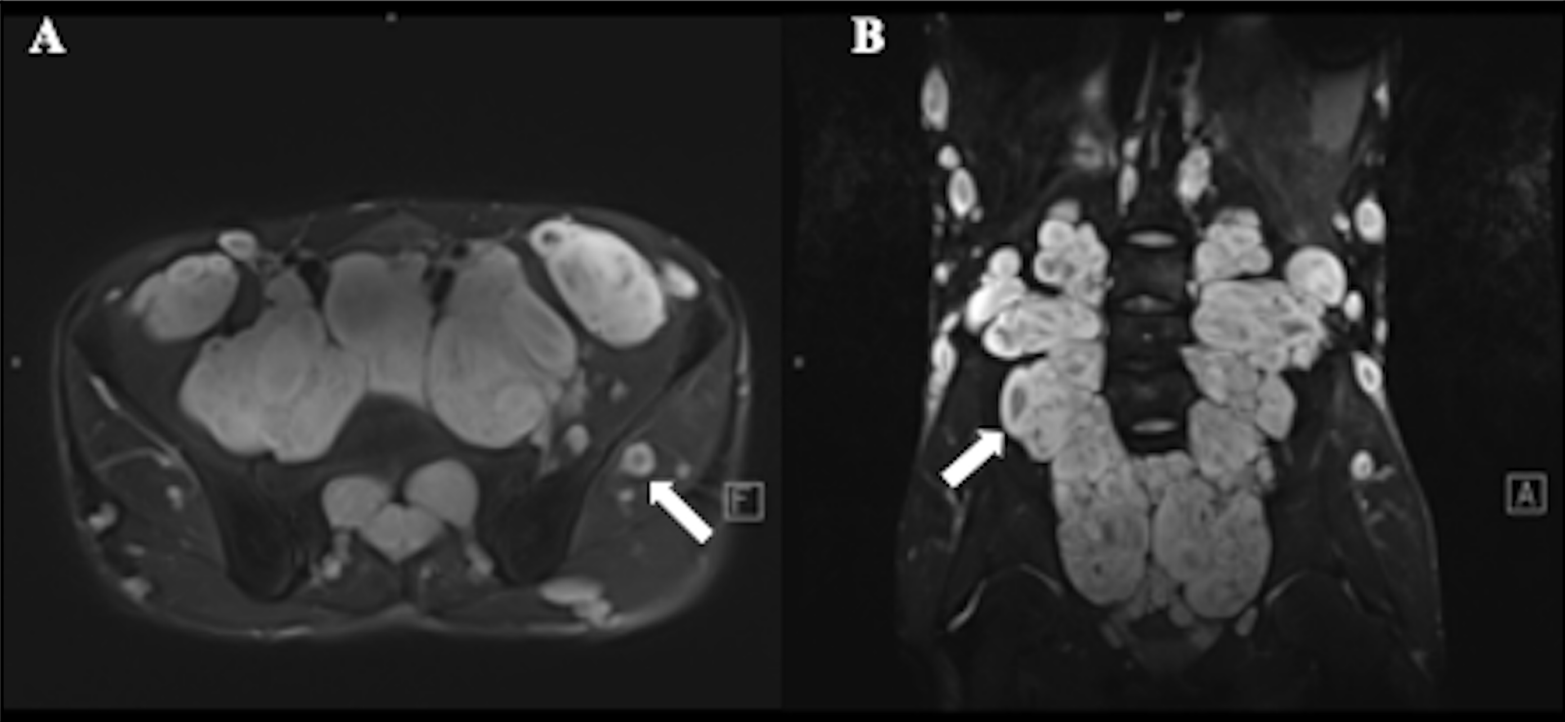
A 17-year-old male with plexiform neurofibroma (PNF) who presented multiple masses throughout the body. T2-weighted MR image showed a target sign (arrow) with peripheral area of high intensity and central area of low intensity. (A) Multiple nodules and lumps in the walking area of the bilateral femoral nerve, sciatic nerve, and obturator nerve; (B) Multiple nodules and lumps in the epidermis, subcutaneous and soft tissues of the abdomen and pelvis.
